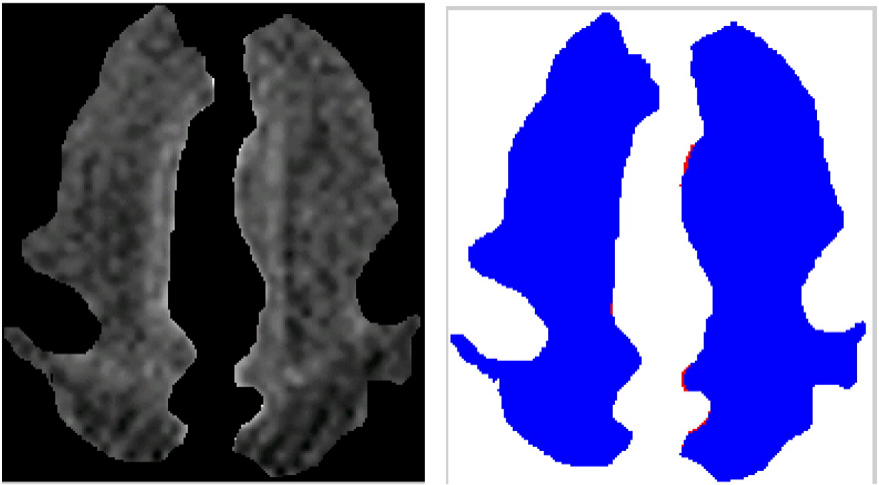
(Left) white matter region with some gray matter on the boundary in a pre-processed MRI slice; and (right) some false positives (in red) detected on the boundaries by our stochastic algorithm.
